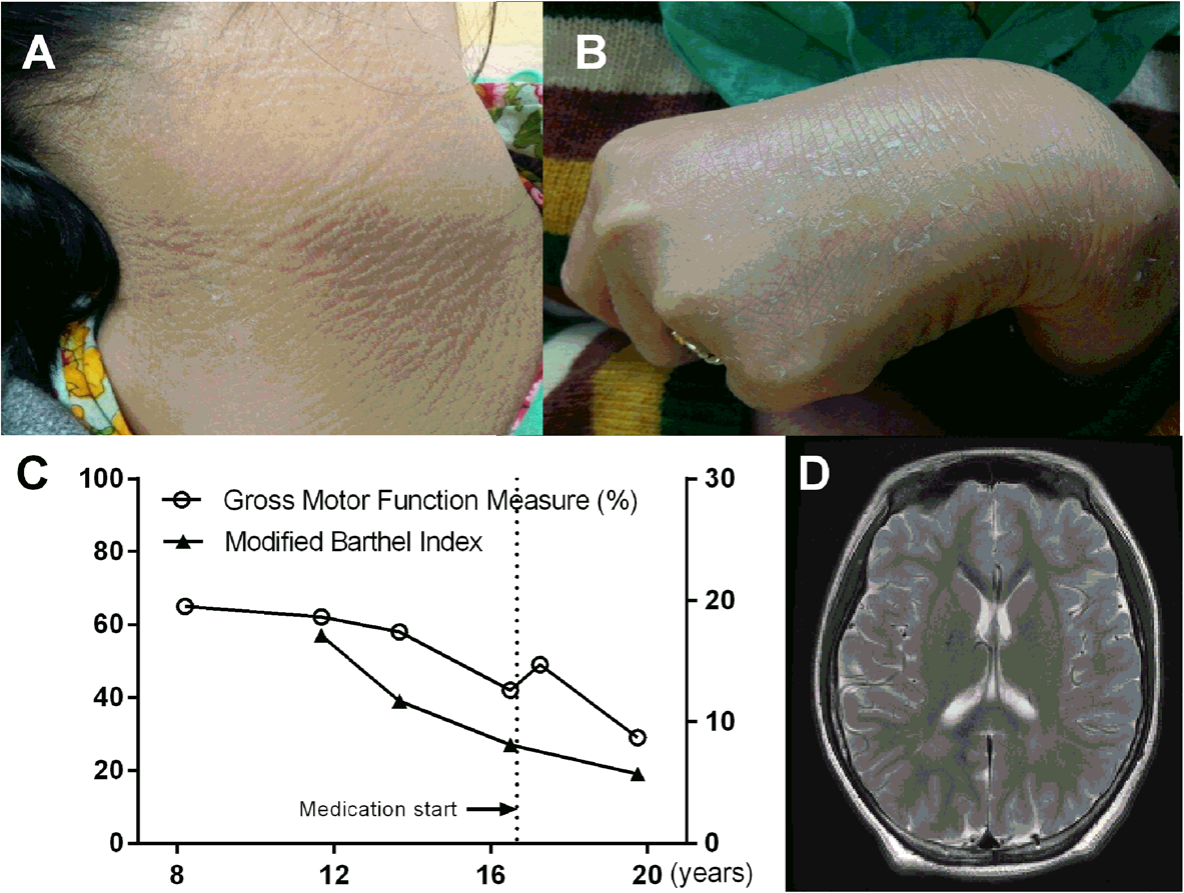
Photographs of ichthyosis on the (a) posterior neck and (b) right elbow. c Graph depicting progressive deterioration in domains of gross motor function based on the percentage score achieved in the Gross Motor Function Measure (GMFM) and modified Barthel Index. The dashed vertical line indicates the time of initiating oral medication for dystonia and tremor. d Brain MRI performed at 13 years of age showing nonspecific T2 hyper-intensities at bilateral parietal deep white matter
